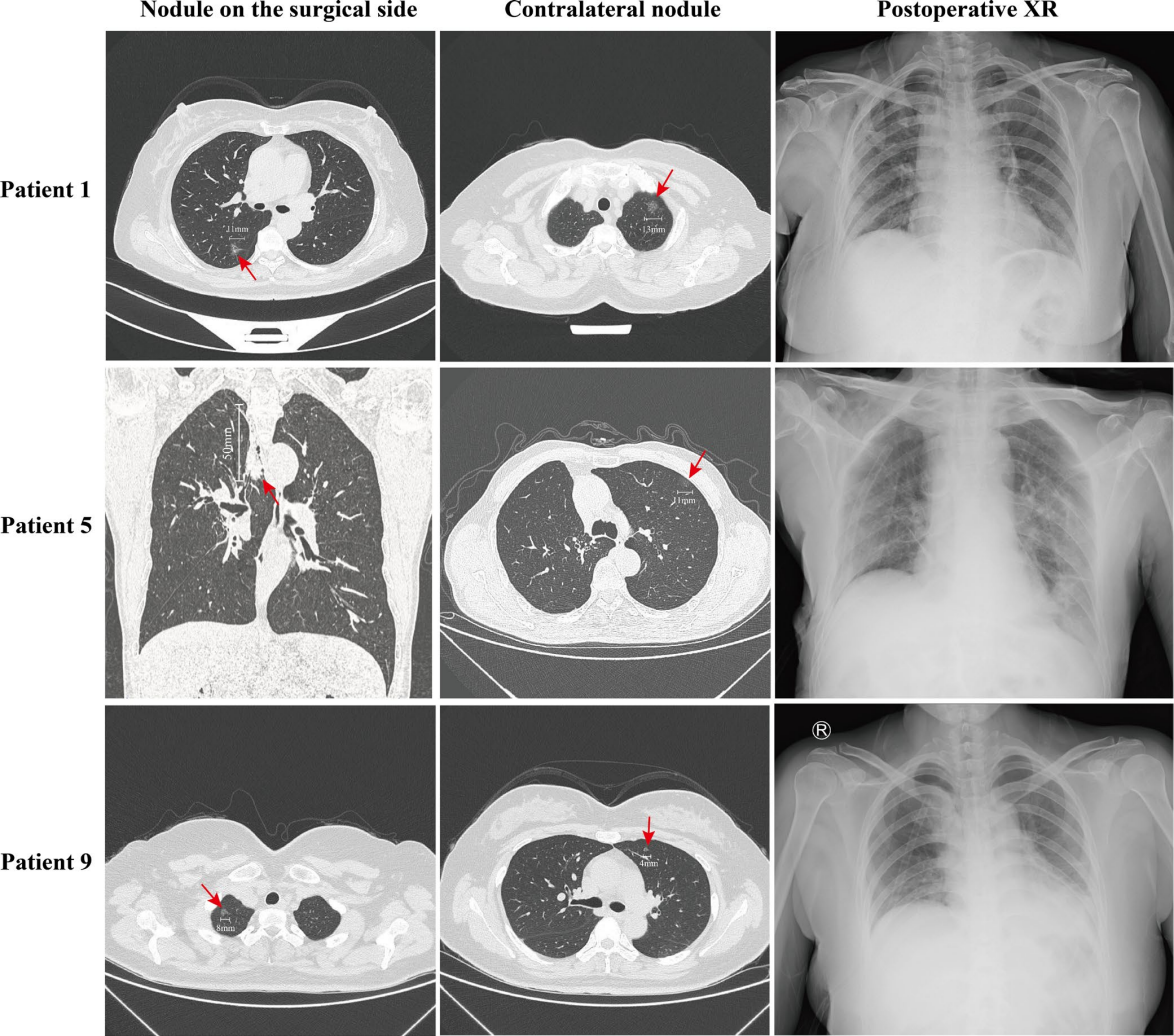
Typical imaging pictures of bilateral pulmonary nodules and postoperative anteroposterior chest radiographs of patient 1, patient 5, and patient 9. (XR, X‐radiography).
